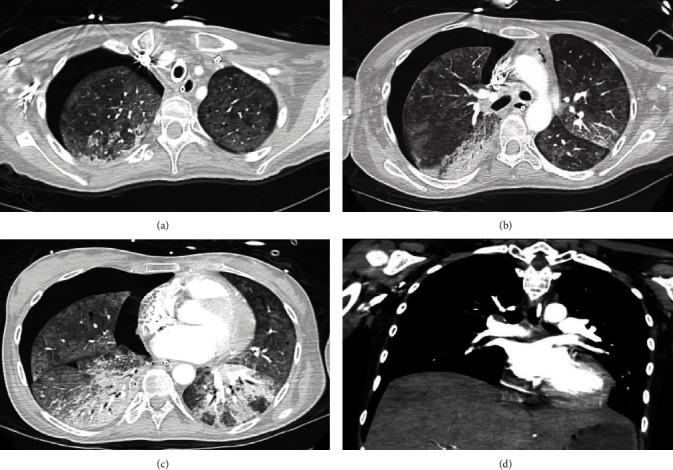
Computed tomography of the Chest. (a–c) Large right pneumothorax with small pneumomediastinum and mediastinal shift compatible with tension pneumothorax (unidentified on daily CXR). Diffuse ground-glass opacities with dense consolidations in both inferior lobes, early fibrosis in the right middle lobe, and multiple pneumatoceles. (d) Acute segmental pulmonary embolism is noted in the posterior segment of the right lower lobe.
